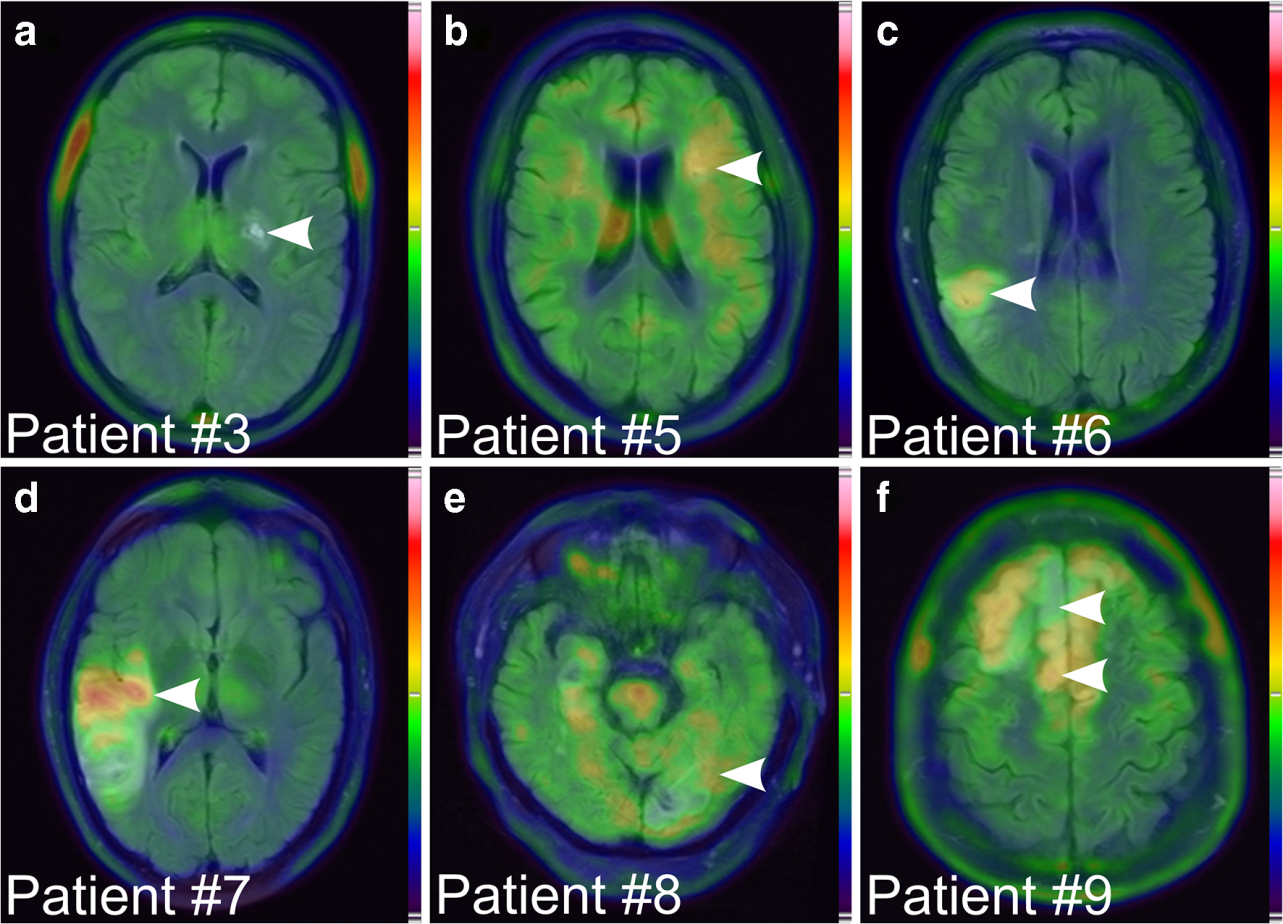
FLAIR/PET overlay of patient #3 (PACNS), #5 (lymphocytic vasculitis), #6, #7, #8 (stroke of non-vasculitis etiology), and #9 (moyamoya) (a–f, respectively). Arrows highlight the dominant lesions
